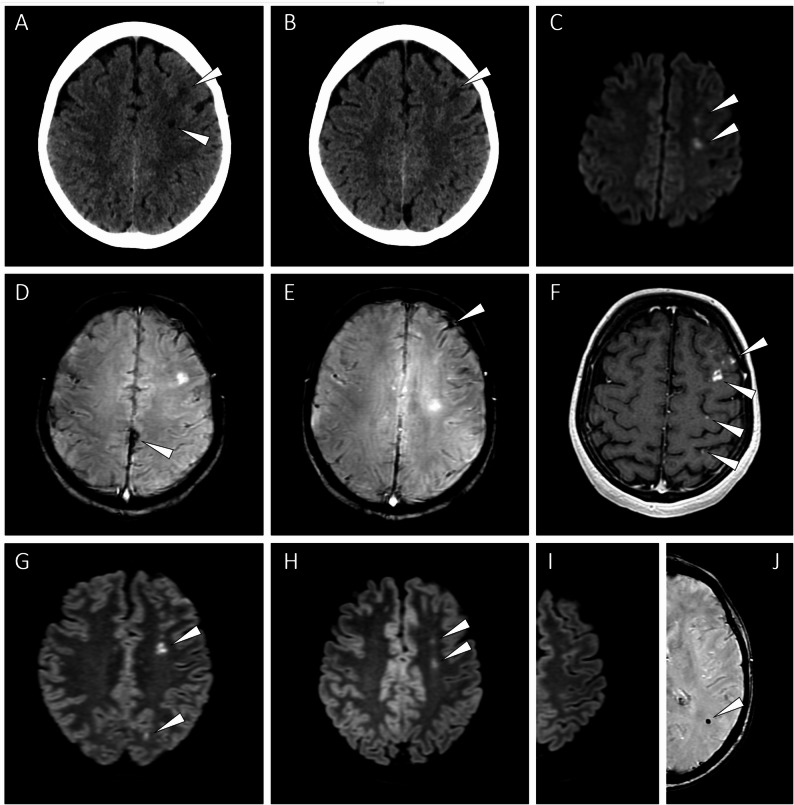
Follow-up CT and MRI scans. Subacute subcortical and cortical hypodensities in the left-sided anterior border zone, 2 days after presentation (A, B). Axial DWI MRI confirming acute ischaemia in the subcortical alterations of the left frontal lobe, 7 days after presentation (C). Representative images of axial SWI MRI confirming the cSAHs (D, E). Axial post-contrast T1 MRI showing luxury perfusion in the ischaemic lesions (F). Axial FLAIR MRI demonstrating hyperintense lesions consistent with vasogenic oedema (G). Follow-up FLAIR images showing minimal hyperintense lesions consistent with chronic infarcts confined to the subcortical alterations with initial DWI hyperintensity (H). No residual infarcts can be appreciated in the cortical luxury perfusion sites (I). A microbleed appeared in the immediately subcortical white matter of the left parietal lobe on SWI (J). cSAH, convexity subarachnoid haemorrhage; CT, computed tomography; DWI, diffusion-weighted imaging; FLAIR, fluid-attenuated inversion recovery; MRI, magnetic resonance imaging; SWI, susceptibility-weighted imaging
